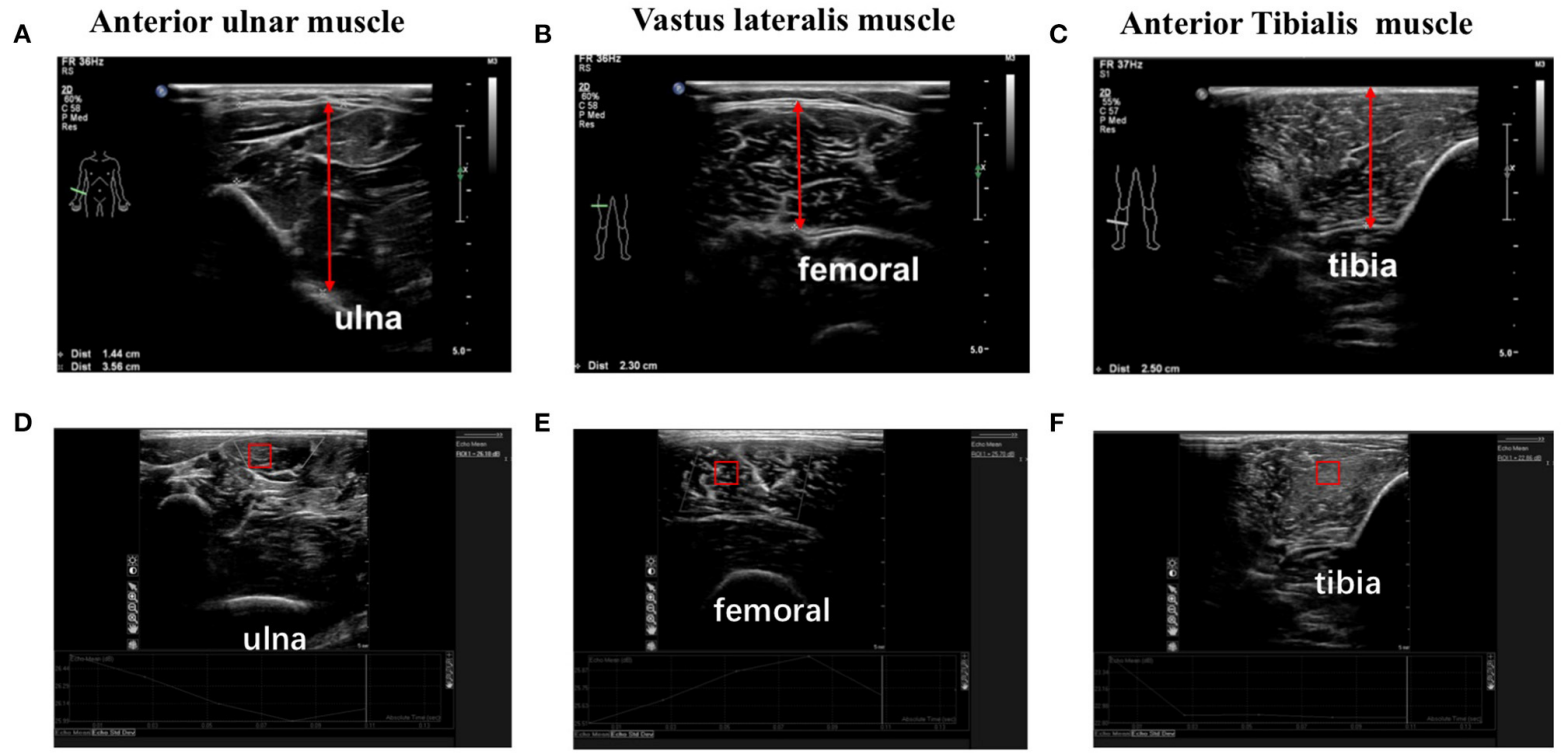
The thickness and quality of anterior ulnar muscle, vastus lateralis muscle and anterior tibialis muscle were detected by ultrasound. (A–C) The thickness of anterior ulnar muscle, vastus lateralis muscle, and anterior tibialis muscle were detected by ultrasound; (D–F) The gray value of anterior ulnar muscle, vastus lateralis muscle and anterior tibialis muscle were analyzed by QLAB software to detect the muscle quality. Square sampling frame represents Region Of Interest (ROI).
